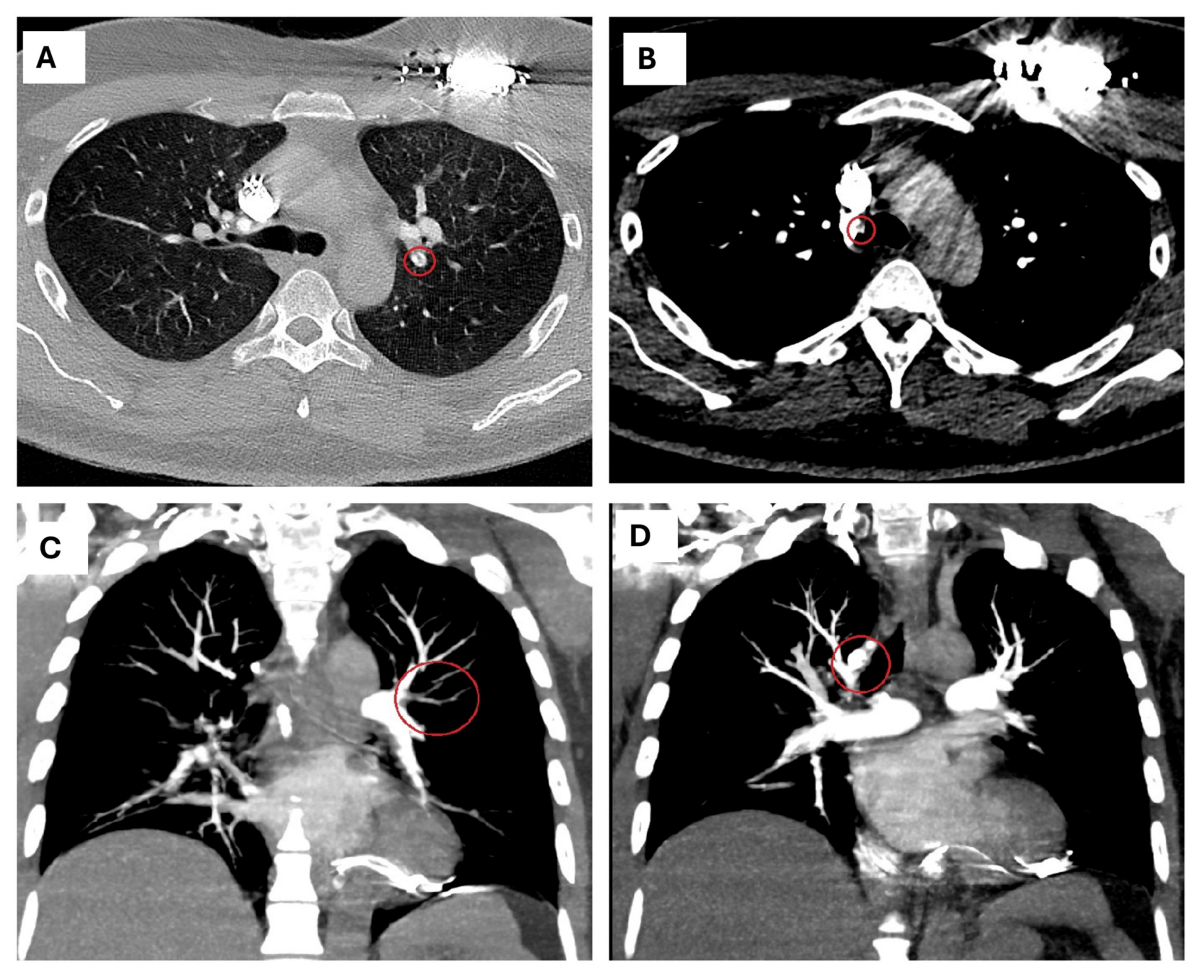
The patient’s computed tomography-pulmonary embolism (CT-PE) protocol scan A) Axial view demonstrating the left upper lobe segmental artery filling defect, encircled in red; B) Axial view demonstrating the right lower lobe segmental artery filling defect, encircled in red; C) Coronal view demonstrating the left upper lobe segmental artery filling defect, encircled in red; D) Coronal view demonstrating the right lower lobe segmental artery filling defect, encircled in red.
